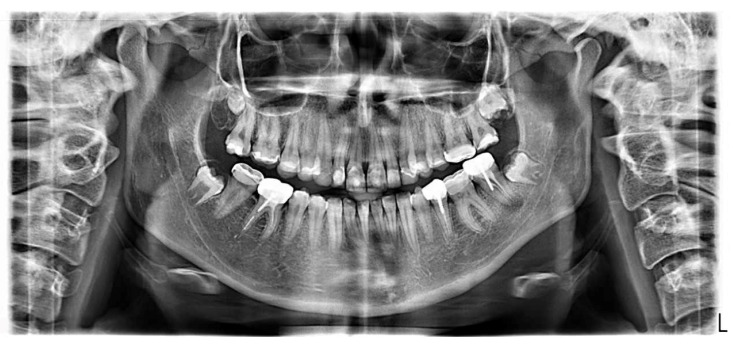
Panoramic radiograph at 8-month follow-up after re-treatment on 47.
